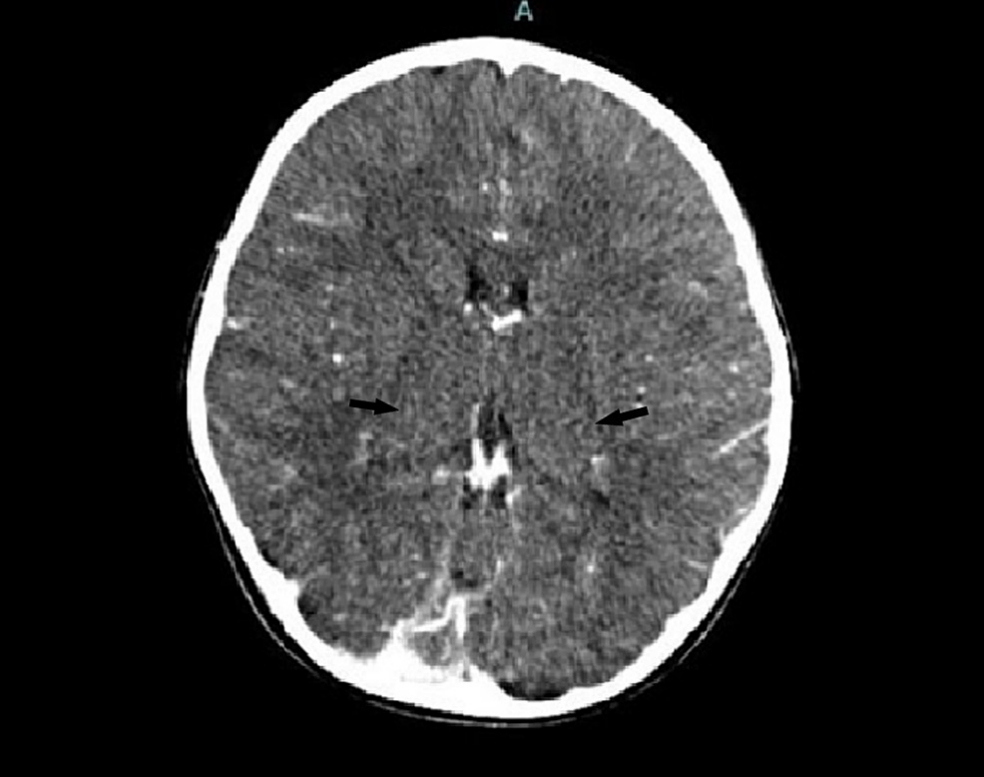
Contrasted CT brain shows bulky bilateral thalami. No focal lesion. No leptomeningeal enhancement.
